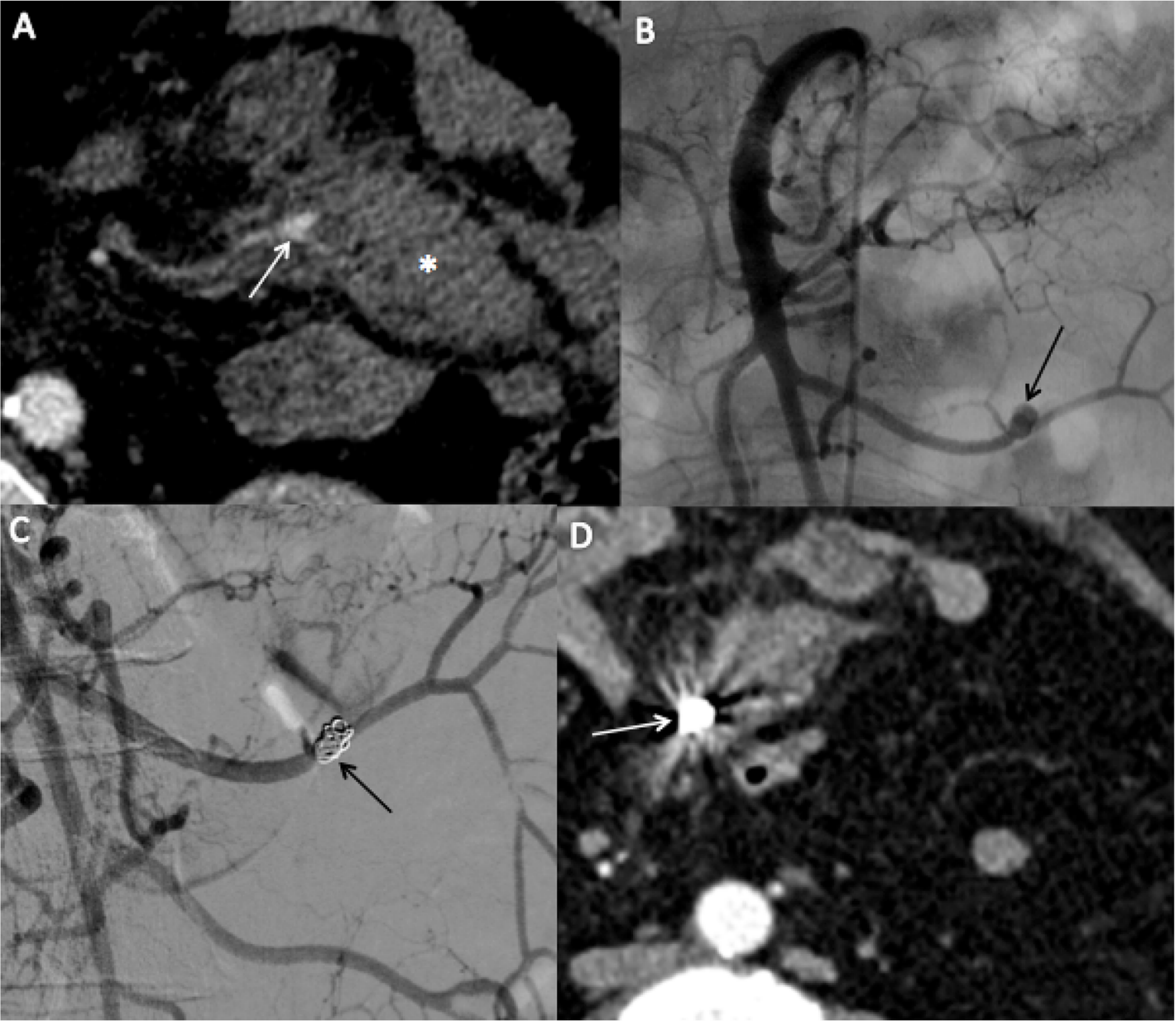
A 67-year-old female with blunt trauma. Axial CT scan (A) shows a mesenteric haematoma (*) associated with irregularity of a branch of the superior mesenteric artery, suggestive of pseudoaneurysm (white arrow). Angiography after catheterization of the SMA (B) confirmed the presence of a pseudoaneurysm (black arrow). After TAE using two fibered coils, the pseudoaneurysm is no longer filled (black arrow), and the feeding artery remains patent (C). A follow-up CT scan performed two days after embolization confirms the absence of filling of the pseudoaneurysm (white arrow) and the reduction in size of the haematoma (D)
